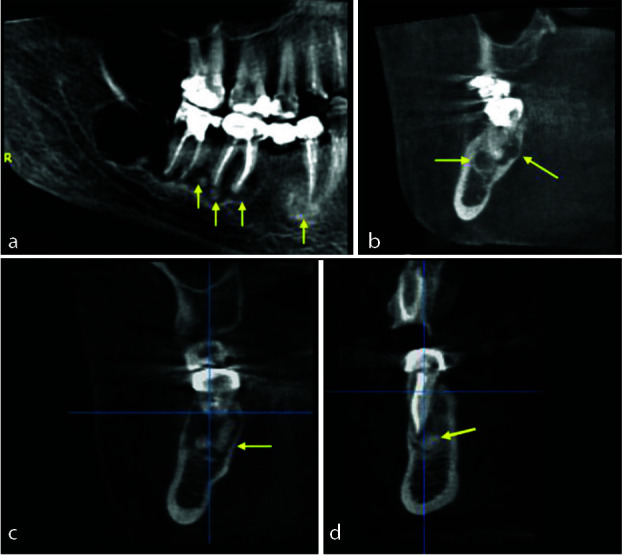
a: Panoramic reconstruction exhibiting the periapical lesions located in apices of the left mandibular, b: Paraxial viewing the lesions associated with the second molar, c: Paraxial presenting the lesions associated with the first molar, d: Paraxial exposing the lesions associated with the first premolar
